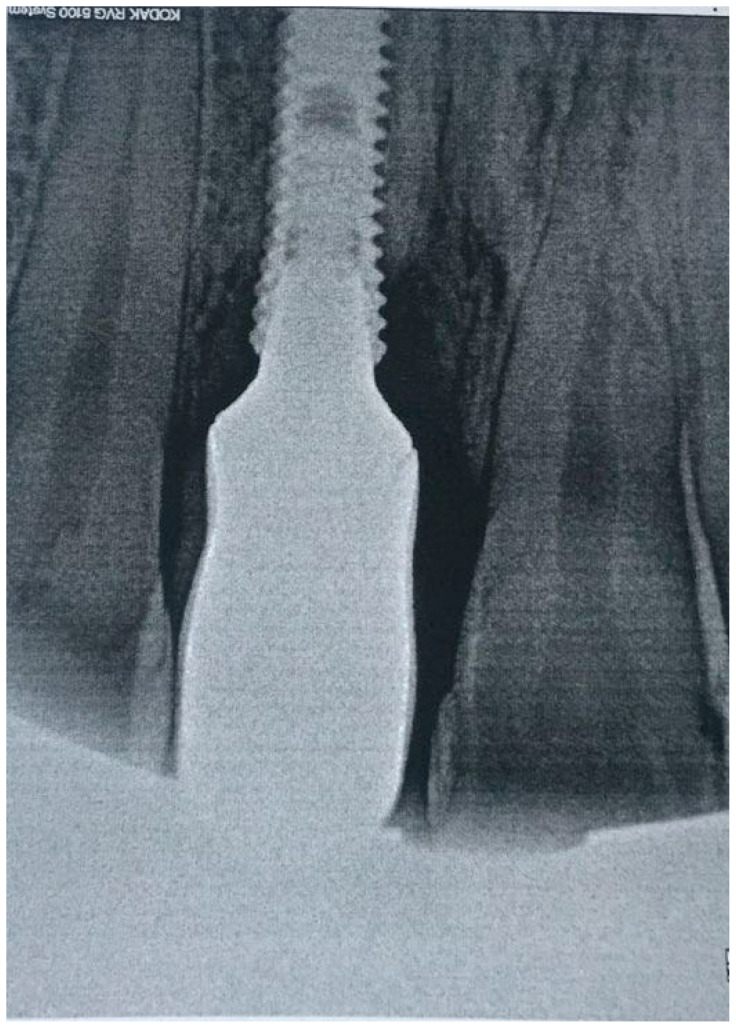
X-ray of patient before treatment in position 1.1 of dental implant.
